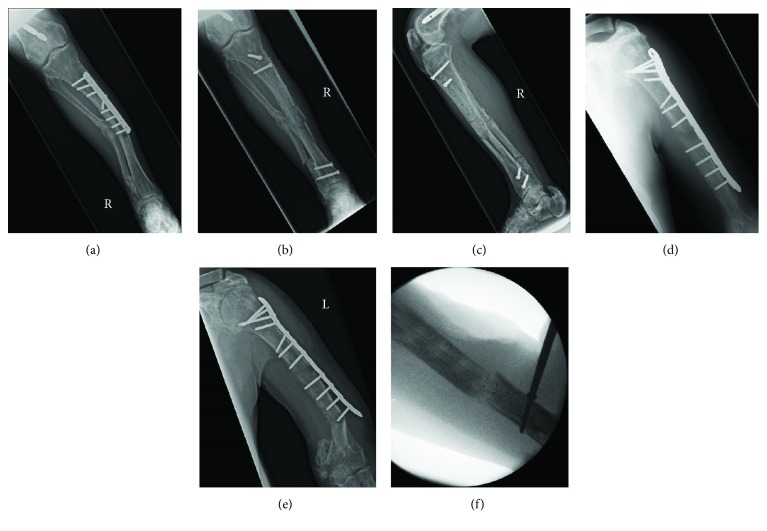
(a) Preoperative AP lower leg X-ray showing a periprosthetic fracture at the distal aspect of the metal plate with valgus deformity. (b, c) AP and lateral lower leg X-rays showing the stabilisation and restoration of alignment of the long bone with a tibial CFR-PEEK nail using proximal and distal locking screws. (d, e) Preoperative AP humerus X-rays showing failure of the metal plate and adjacent bone over time. (f) Intraoperative fluoroscopy image showing stabilisation of the long bone with a humeral CFR-PEEK nail.
